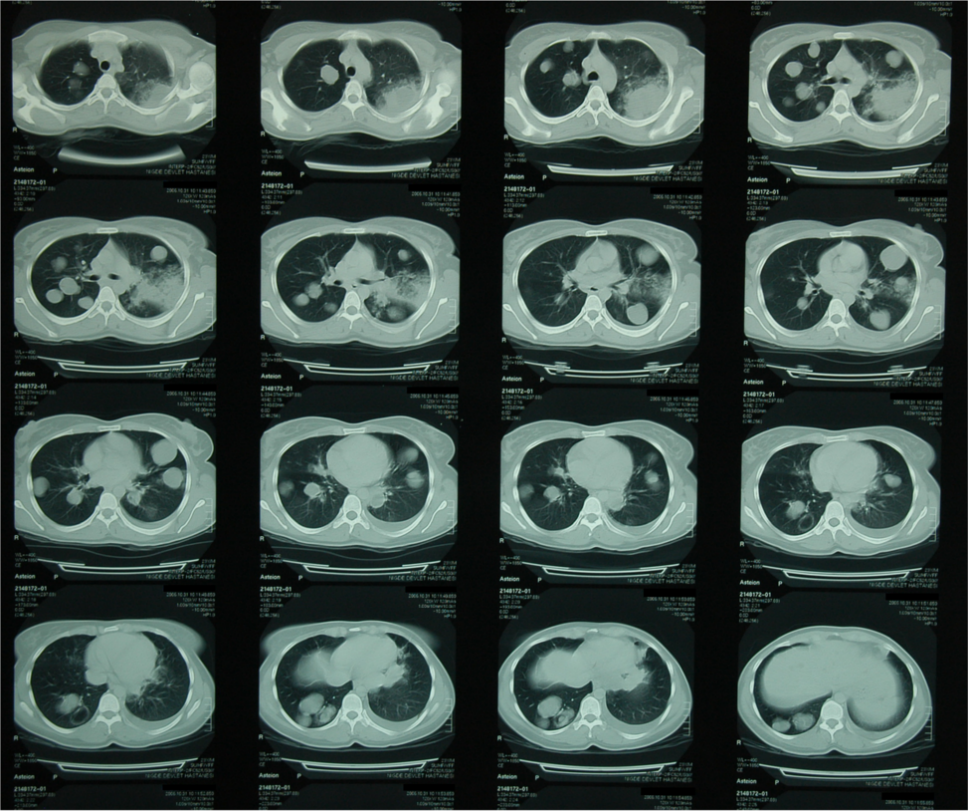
Computed tomography scan demonstrates bilateral multiple lung cysts.
